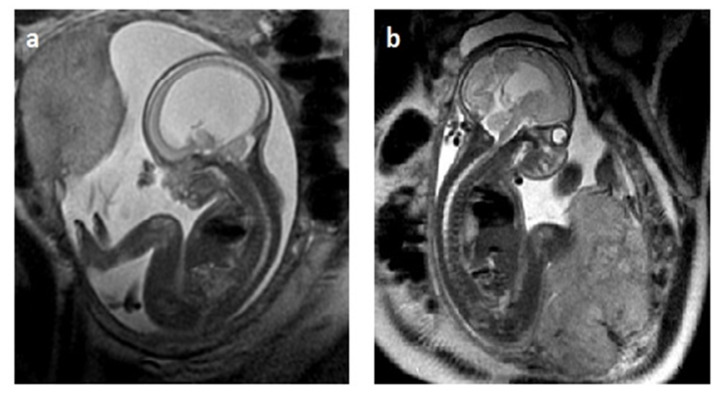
A comparison of the fetal MRI in the sagittal plane, depicting the change in the lateral ventricular size of the fetal brain (a) pre-VAVI vs. (b) post-VAVI [99].
